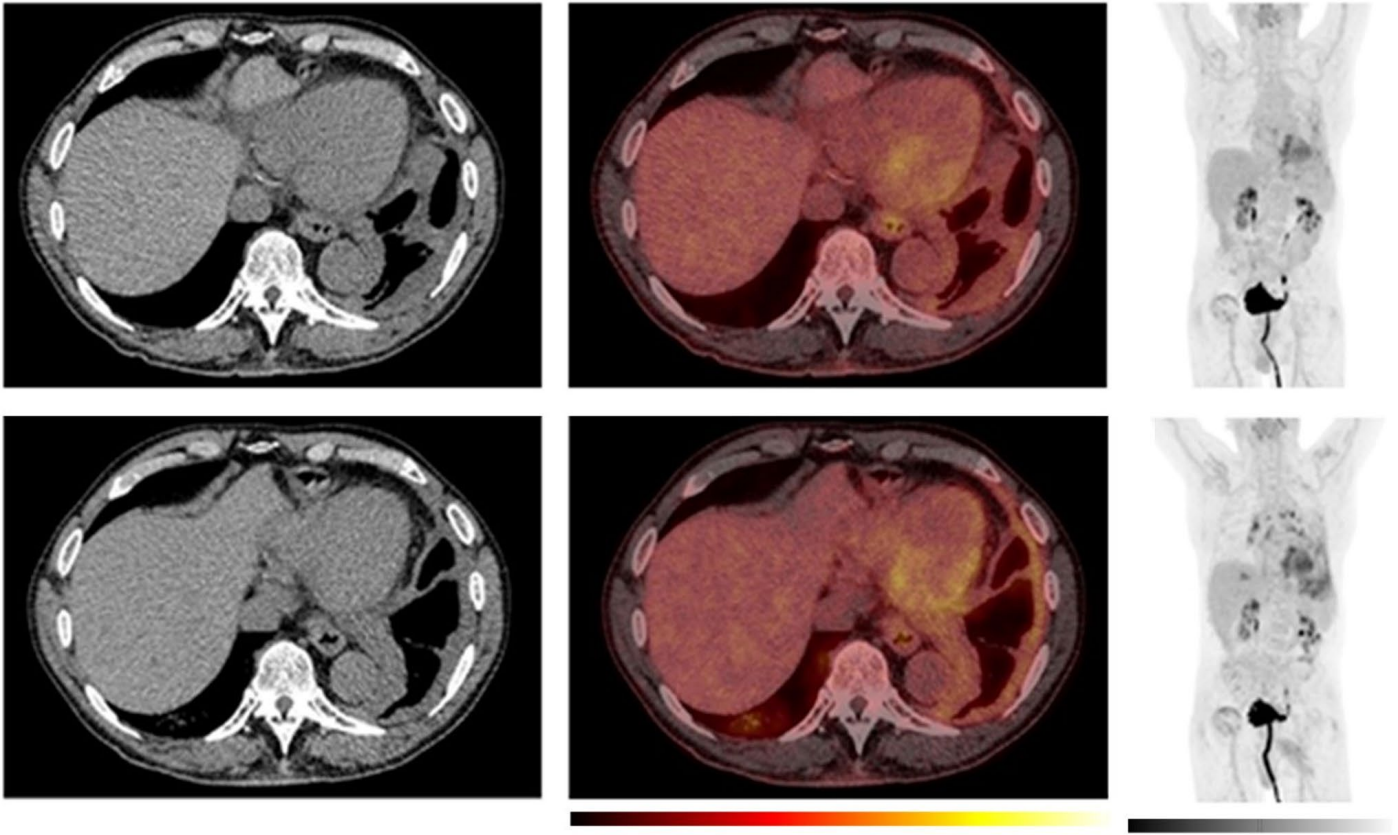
Example of a patient with an initial increase in MTV and [^18^F]fluorodeoxyglucose (FDG) uptake despite long-term objective tumour response/disease control. Baseline (top row) and week-5 (bottom row). To the left, axial computer tomography (CT) images, in the middle, axial positron emission tomography (PET)/CT images and to the right the maximum intensity projection (MIP). The PET images are observed within a 0-10 SUV window. While the patient had stable disease according to the modified Response Criteria in Solid Tumours (mRECIST) at the first CT response assessment, both metabolic tumour volume (MTV) and [^18^F]FDG uptake showed an increase from baseline to week-5. The patient was classified as having a partial response at the week-12 CT scan. The patient demonstrated clinical signs of improvement already at the week-5 visit. The planned one-year PET scan was cancelled due to hip surgery. The treatment was discontinued after 9 months due to the development of severe Immune-mediated thrombocytopenia (ITP) and the patient died of disease progression 2.5 years after the start of the treatment
